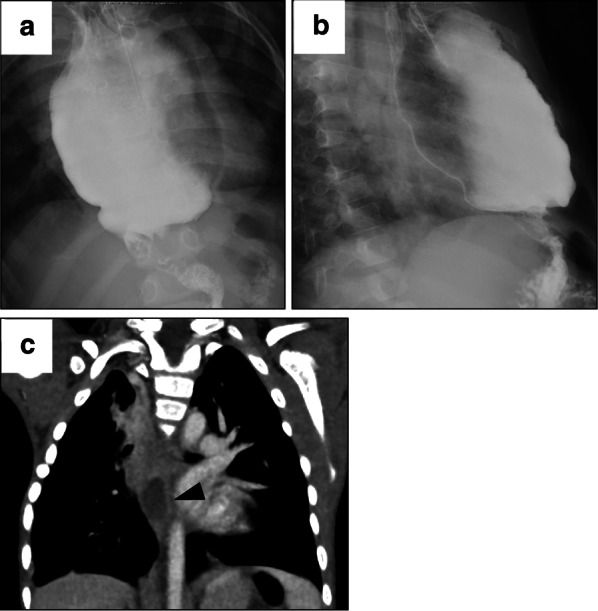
Postoperative course. a, b Frontal and lateral views of the contrast study 6 months after operation. The mobilized stomach is located midline in the mediastinum. c CT scan at 2 years after operation. Mucus pooling is seen in the residual esophagus (black arrowhead)
